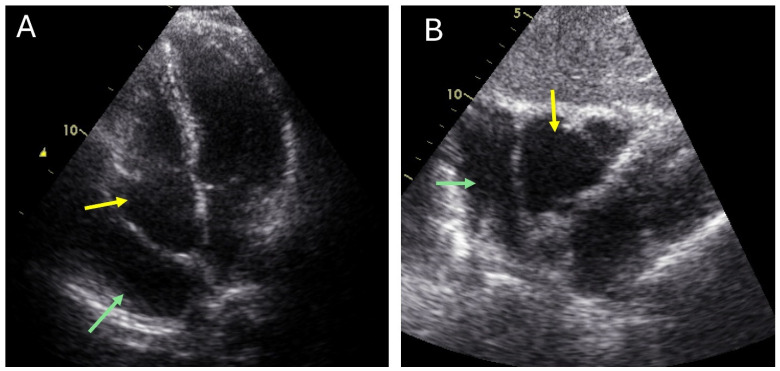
Transthoracic echocardiography of pericardial cyst (green arrow) adjacent to the right atrium (yellow arrow) in (A) Apical 4-chamber view and (B) Subcostal 4-chamber view.
